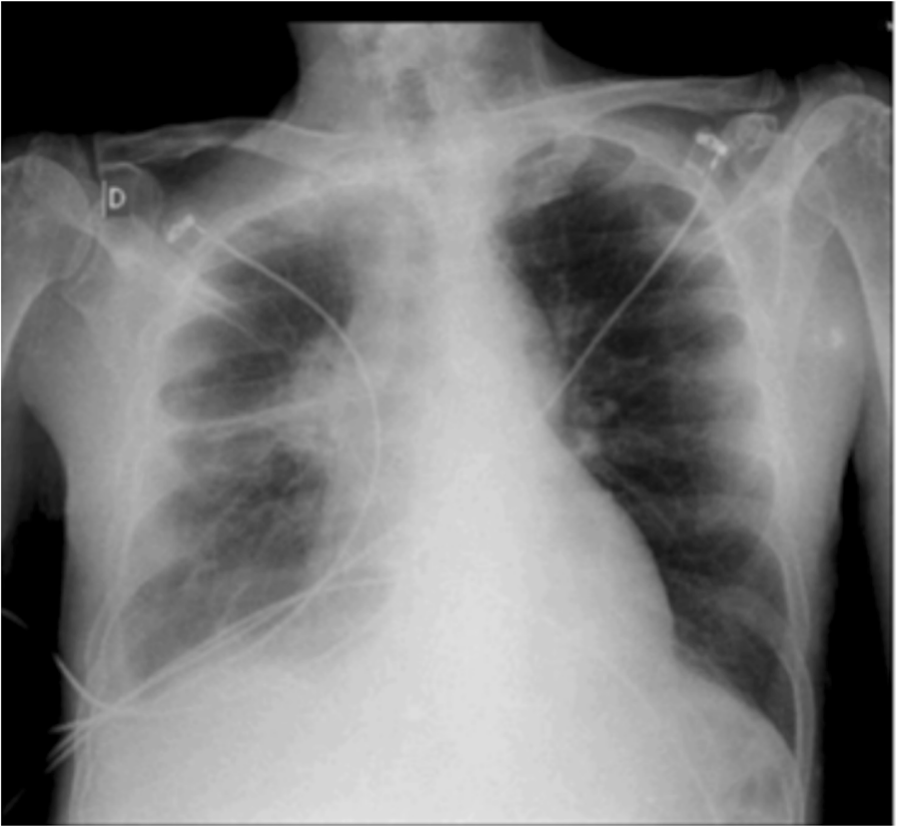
Chest X-ray of patient 2. Chest X-ray performed at in the emergency department showing cardiomegaly. Note the right tumoural lung opacity
